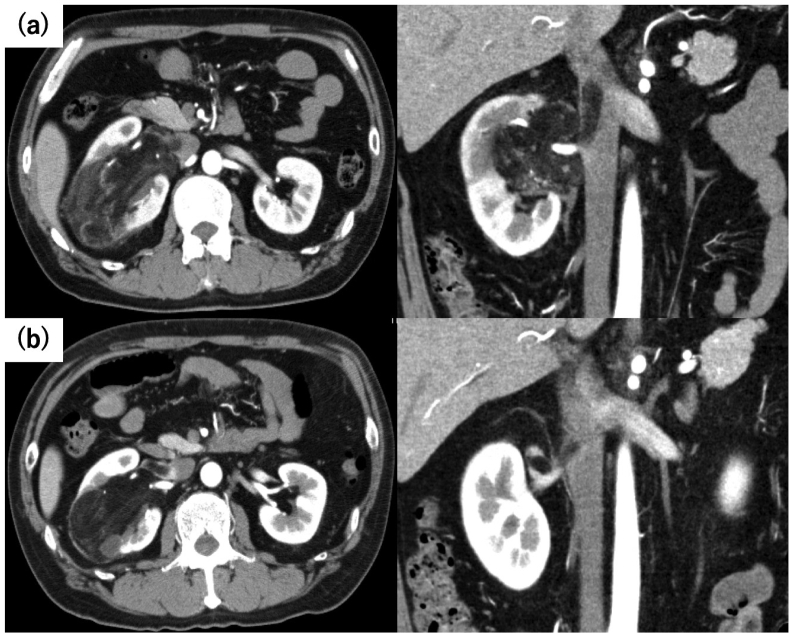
Contrast agent CT scan; Tumor and IVC thrombus (a) Pre-treatment (b) 3 months after everolimus administration.
